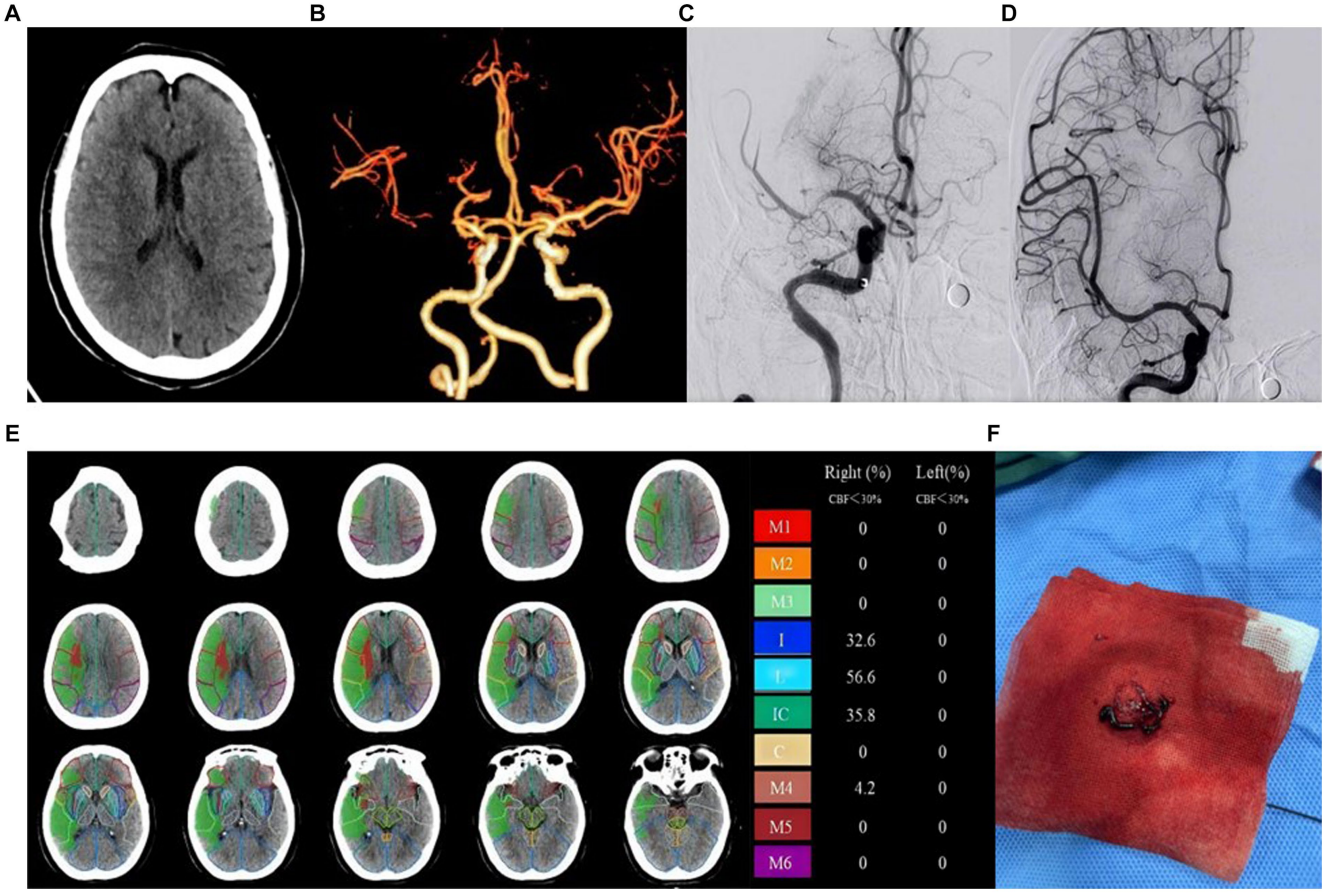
Example of patient with poor prognosis (mRS > 2) and high ASPECT scores. Female, 73 years old, woke up with limb weakness for 6 hours. ASPECTS is 6 points. (A) NCCT shows patchy hypodensity in the right cerebral hemisphere. (B) CTA MIP shows occlusion of the right middle cerebral artery. (C) DSA shows occlusion of the right middle cerebral artery. (D) DSA shows recanalization of the right middle cerebral artery. (E) The core infarction involves the insula, lentiform nucleus, internal capsule, and M4, along with their respective infarction percentages. (F) Operation sample.
